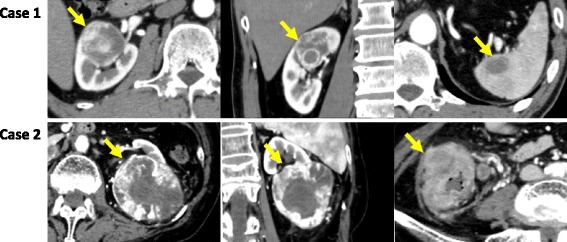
Enhanced computed tomography (CT) showing renal tumor and suspected metastatic tumor or concurrent colon cancer. Above, well-enhanced right renal tumor and splenic tumor of Case 1.Below, large enhanced left renal tumor and ascending colon tumor with lymph node swelling of Case 2
